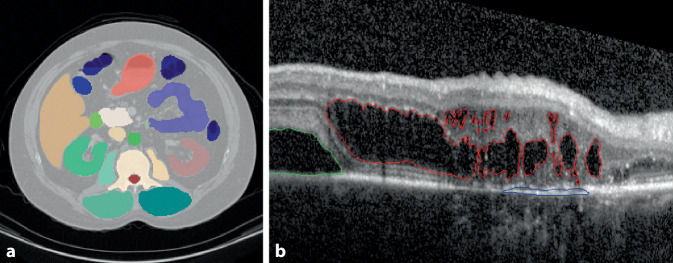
Segmentierungsbeispiele für verschiedene medizinische Anwendungsbereiche. a Segmentierung verschiedener Organe im Computertomografie-(CT-)Bild [48]. b Segmentierung pathologischer Strukturen in der optischen Kohärenztomografie (OCT) der Retina
